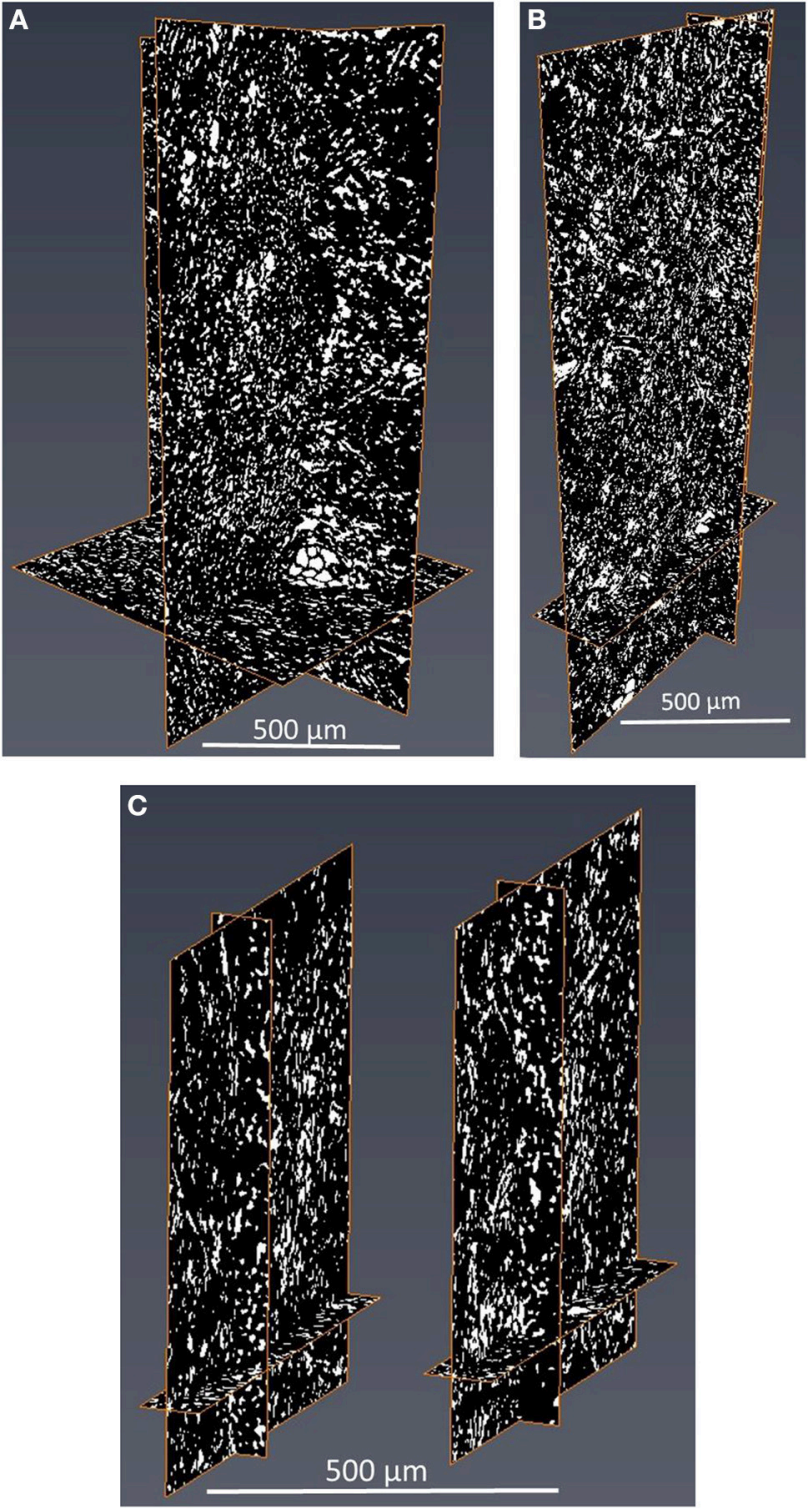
3D X-ray images of (A) 400 gsm sample (B) 200 gsm sample and (C) 100 gsm sample presented with some of layers with intersection of three different planes.
